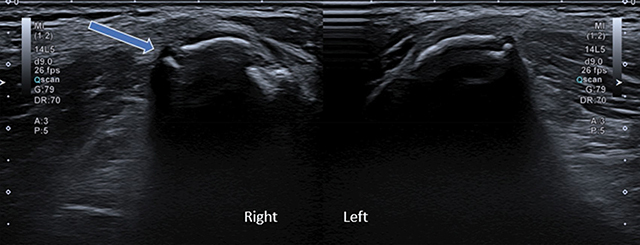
Ultrasound shows an irregular shape and displacement of the ossification center of the right iliac crest.
